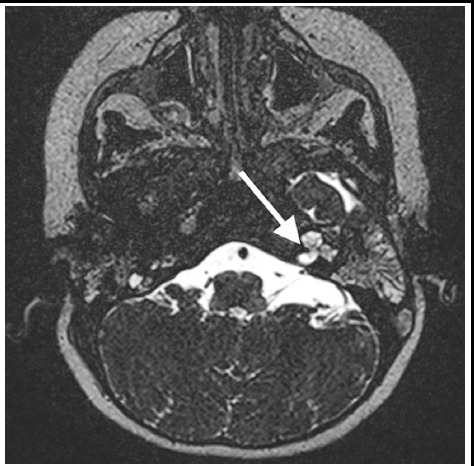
Thin-slice axial coronal computed tomographic image of a sample case with inner ear malformation obtained before cochlear implantation surgery, displays cochlea, and vestibulum in a common cavity containing inner ear fluid.
